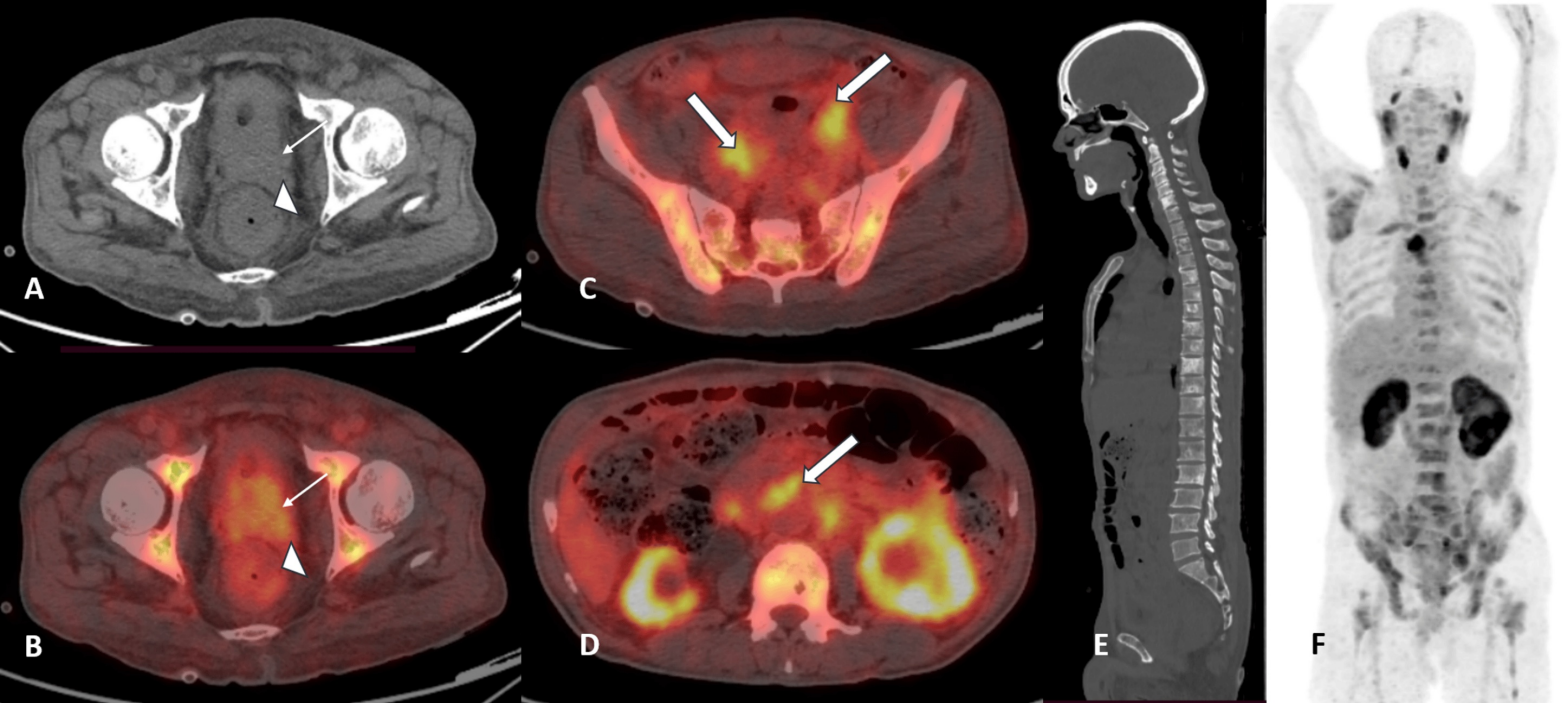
Ga-68 PSMA PET-CT images Trans-axial section views of non-contrast CT (A) and fused Ga-68 PSMA PET-CT images (B-D) showing prostatic primary lesion infiltrating the bladder and urinary bladder wall with low to intermediate PSMA expression (thin arrows in A and B). Also, circumferential mural thickening was noted in the rectum with low-grade PSMA expression (arrowheads in A and B). Fat planes between prostatic and rectal lesions are maintained. Multiple pelvic and retroperitoneal lymph nodes were noted with intermediate- to high-grade PSMA expression (thick arrows in C and D). Maximum intensity projection images (F) showing multiple intermediate to high PSMA-expressing skeletal lesions, with the highest PSMA expression in the sternal lesion. Sagittal section of non-contrast CT images (E) showing multiple mixed lytic and sclerotic skeletal lesions (predominantly sclerotic) in the visualized axial skeleton. Ga-68 PSMA PET-CT: gallium-68-labeled PSMA positron emission tomography-computed tomography
